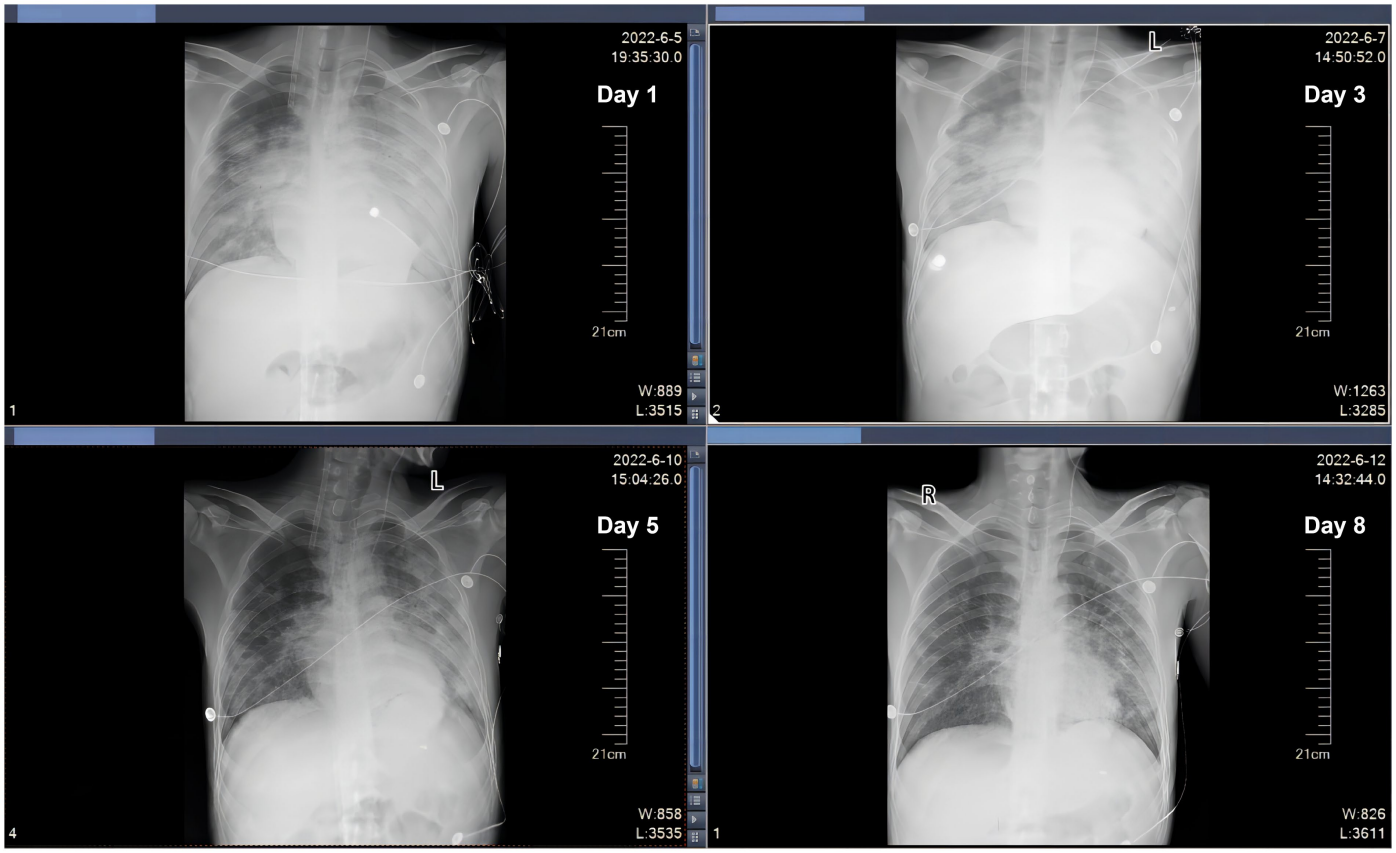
Imaging features of chest X-ray (D1, D3, D5, and D8).
